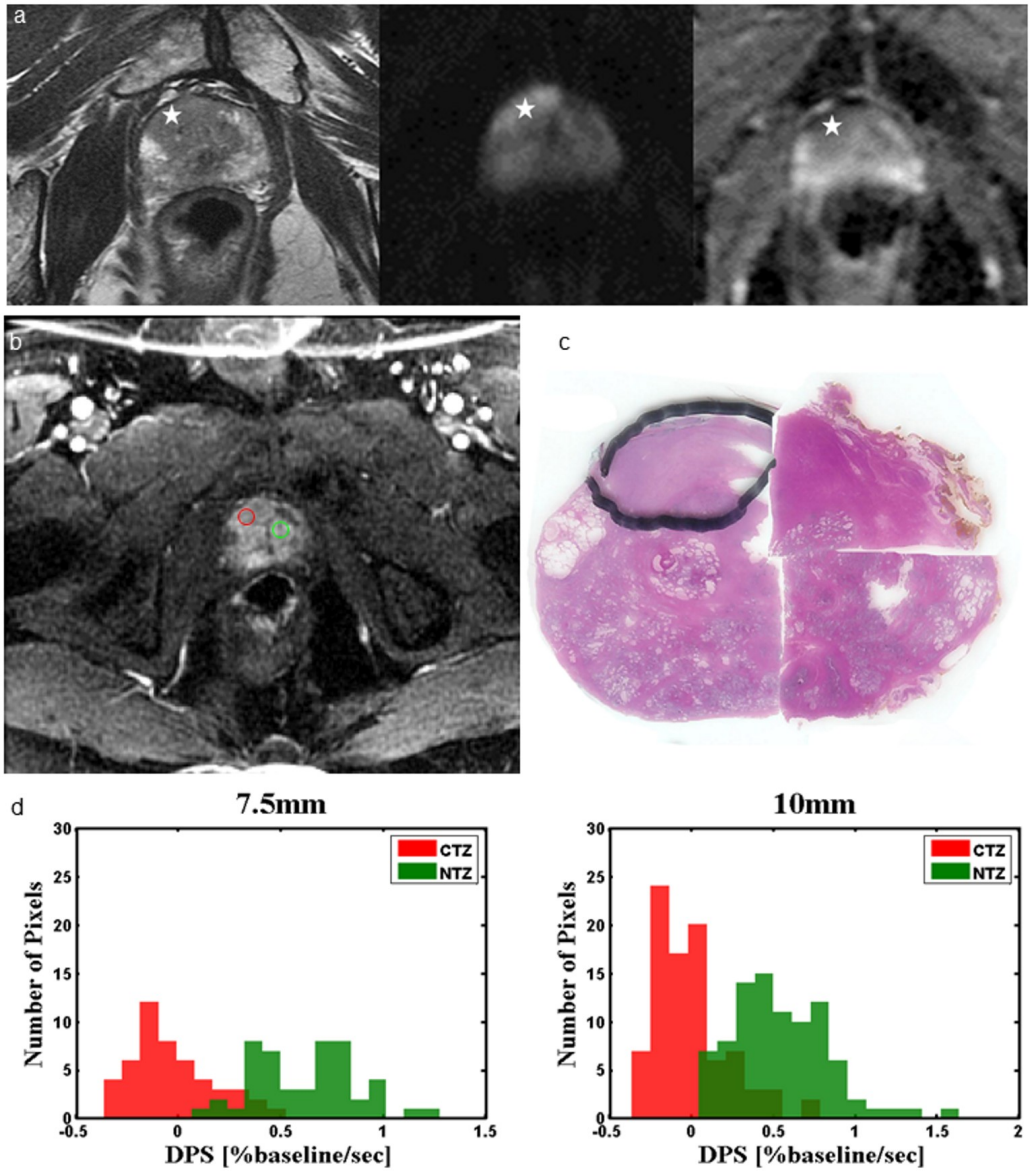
Example of the selected ROI. A 57-year-old patient with elevated PSA levels and previous negative systematic TRUS biopsy. (a) 3T mp-MRI (from left to right: T_2_-weighted fast spin-echo imaging, DWI, and ADC map) demonstrates an area suspected to be cancerous (stars), which was confirmed by performing targeted TRUS biopsy. (b) ROI selection was performed on the basis of corresponding DCE-MRI for CTZ (red circle) and NTZ (green circle). (c) The pathological slice at the corresponding level was correlated to confirm the representativeness of the selected ROI (circled by a marker). (d) The histogram at different diameters of the ROI of CTZ and NTZ.
